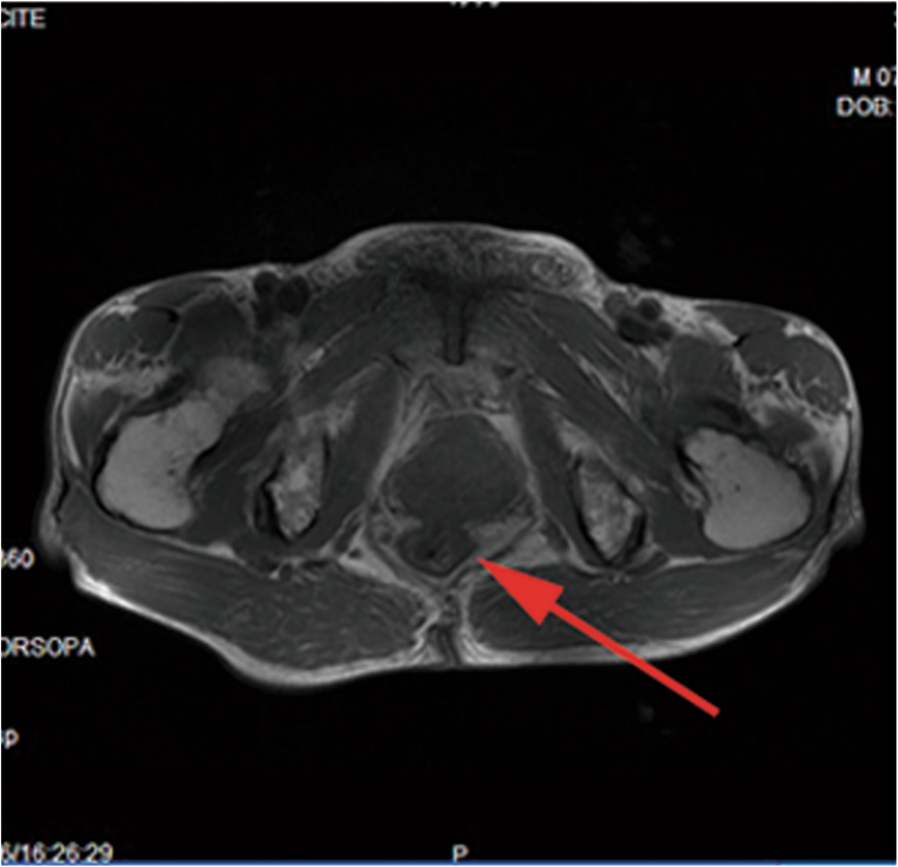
Pelvic enhanced magnetic resonance imaging: a rectal lesion at a distance from the anus of about 86 mm (red arrow).
